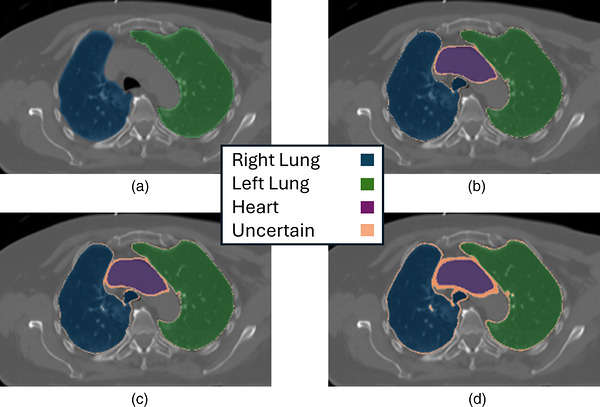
Visualization of reference standard contours (a) and conformal prediction sets overlayed on the original CT image. Solid colors represent predictions within the 90% (b), 95% (c), and 99%(d) coverage requirement, while orange pixels represent uncertain pixels. A legend for each structure color is shown. This example shows uncertain regions within this slice for all three structures. The heart is not contoured in (a), while the U‐Net predictions show a heart mask in the image. It is notable that relative to the size of the predicted heart structure, a larger amount of the structure is uncertain in the heart than in either lung. As the conformal prediction requirements become more strict, the relative area of uncertainty in the heart increases faster than in the lungs.
